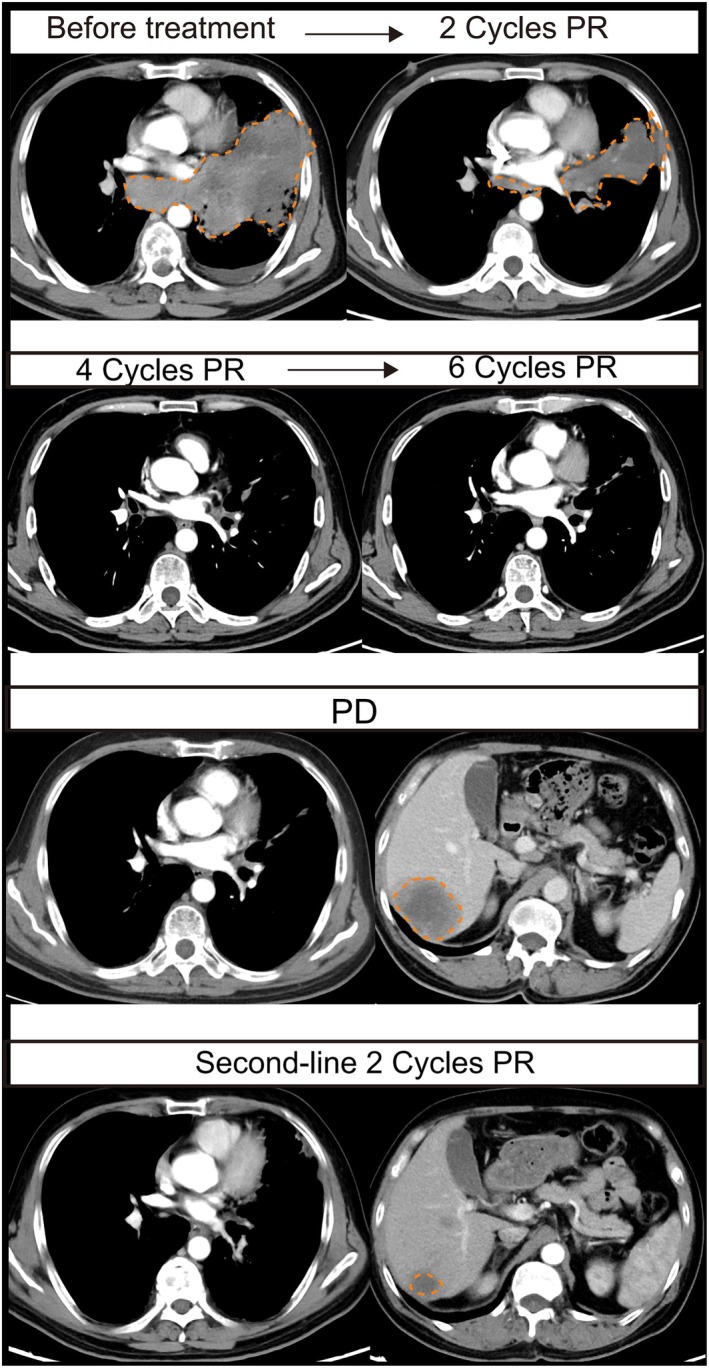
Enhanced CT images of primary tumors and metastatic tumors in the liver highlighted with yellow circles.
